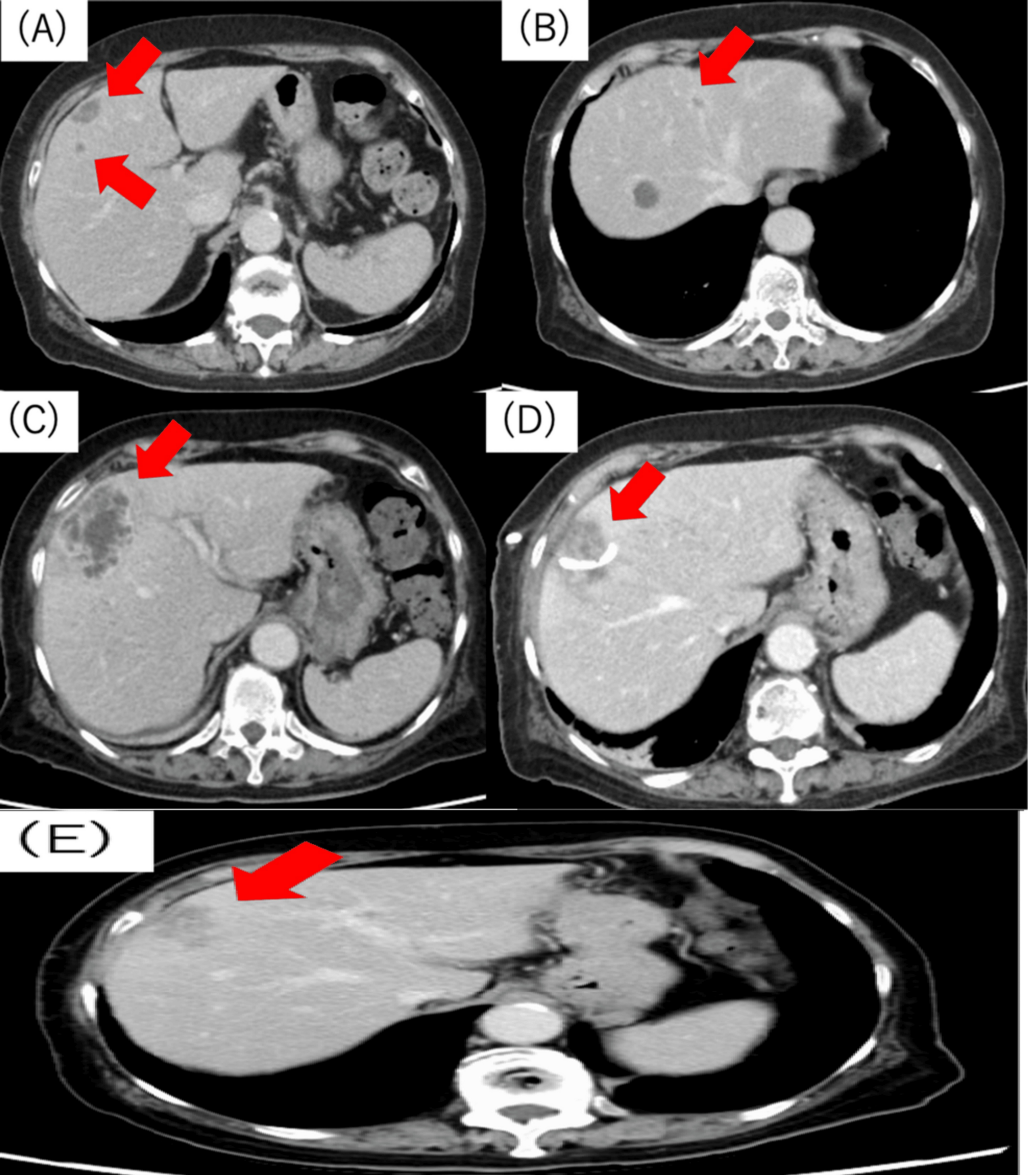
Image of a CT scan taken during readmission and at discharge from the referring hospital. Contrast-enhanced CT showing (A) abscesses in the liver S4 (20 mm) (arrow) and another in (B) S3 (arrow). (C) Antibiotic administration increased the abscess size in S4 (52 mm) (arrow). (D) Percutaneous transhepatic drainage was performed to treat the multifocal abscess in liver S4 (arrow). (E) Despite performing percutaneous transhepatic drainage and providing antibiotic treatment, the abscess remained unresolved at the time of discharge (29 mm) (arrow) CT: computed tomography
